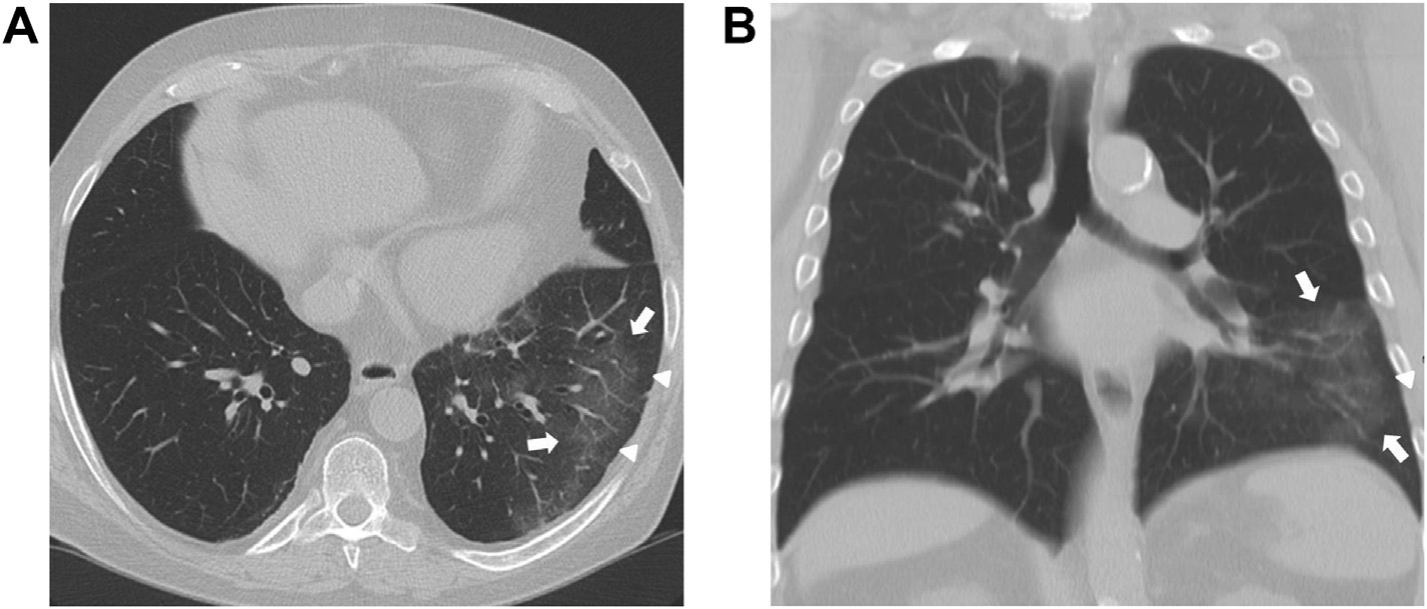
The initial CT scan acquired on the first day of hospitalization showed exclusive left-sided focal ground glass opacity (GGO) in the lower lobe (Figure 1B, coronal plane, *arrows*) with noticeable predominant exclusion of the subpleural space (Figure 1A, transverse plane, *arrowheads*). Associated inter- and intralobular septal thickening and bronchiectasis were present. No consolidation and no signs of lymphadenopathy or pleural effusion were found.
